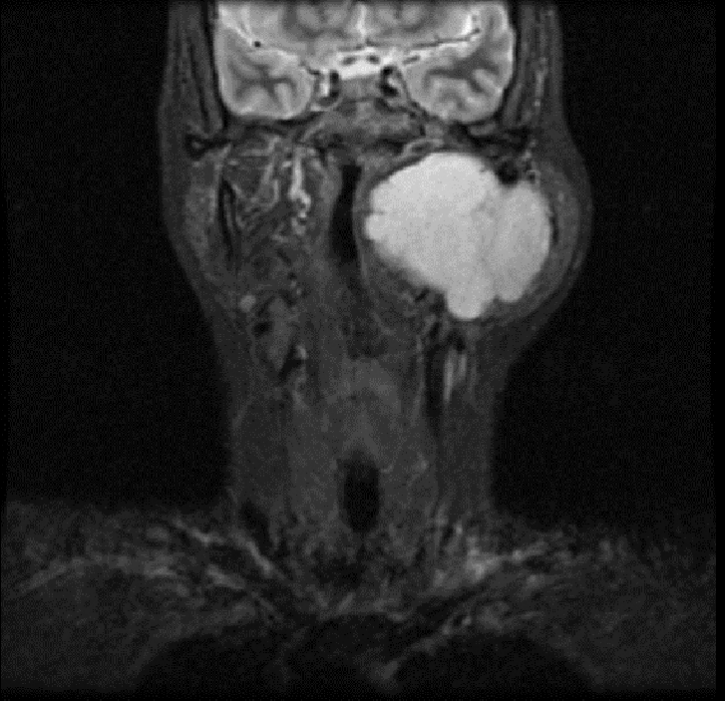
A coronal T2-weighted MRI of the head and neck region (36th week of pregnancy). The left parotid mass is seen distorting the pharynx. The outline of the heterogeneous lesion is clearly demarcated, and tissue planes preserved.
